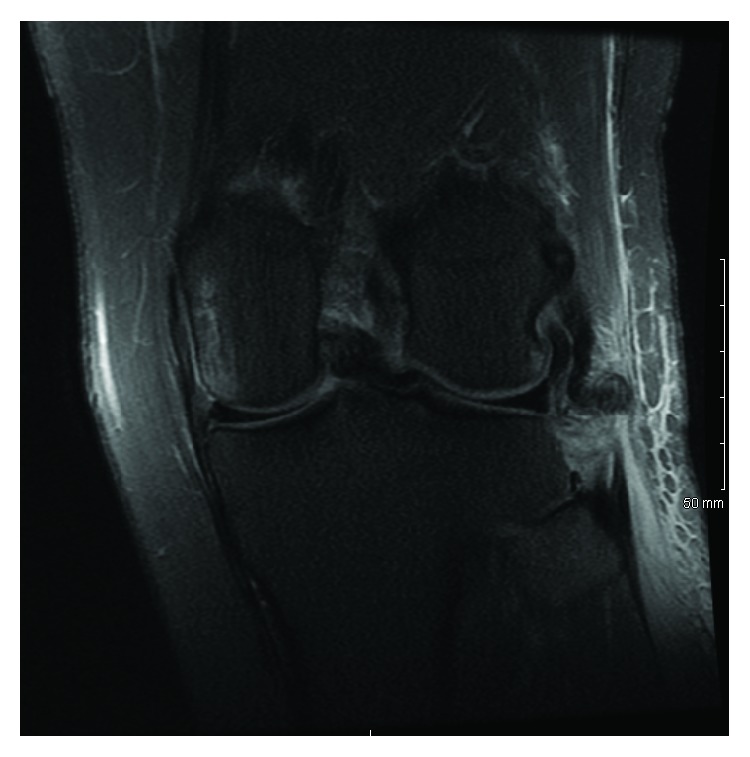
A T2 coronal MRI of the patient's left knee demonstrating a grade III lateral collateral ligament tear with retraction.
